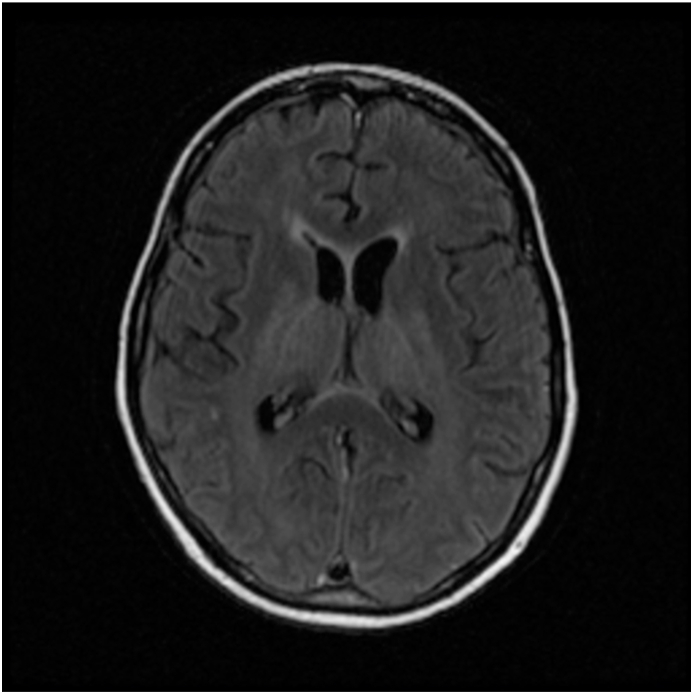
MRI-FLAIR of patient 3; showing hyperintensity in the thalami and the posterior limbs of the internal capsules bilaterally. FLAIR: Fluid-Attenuated Inversion Recovery.
